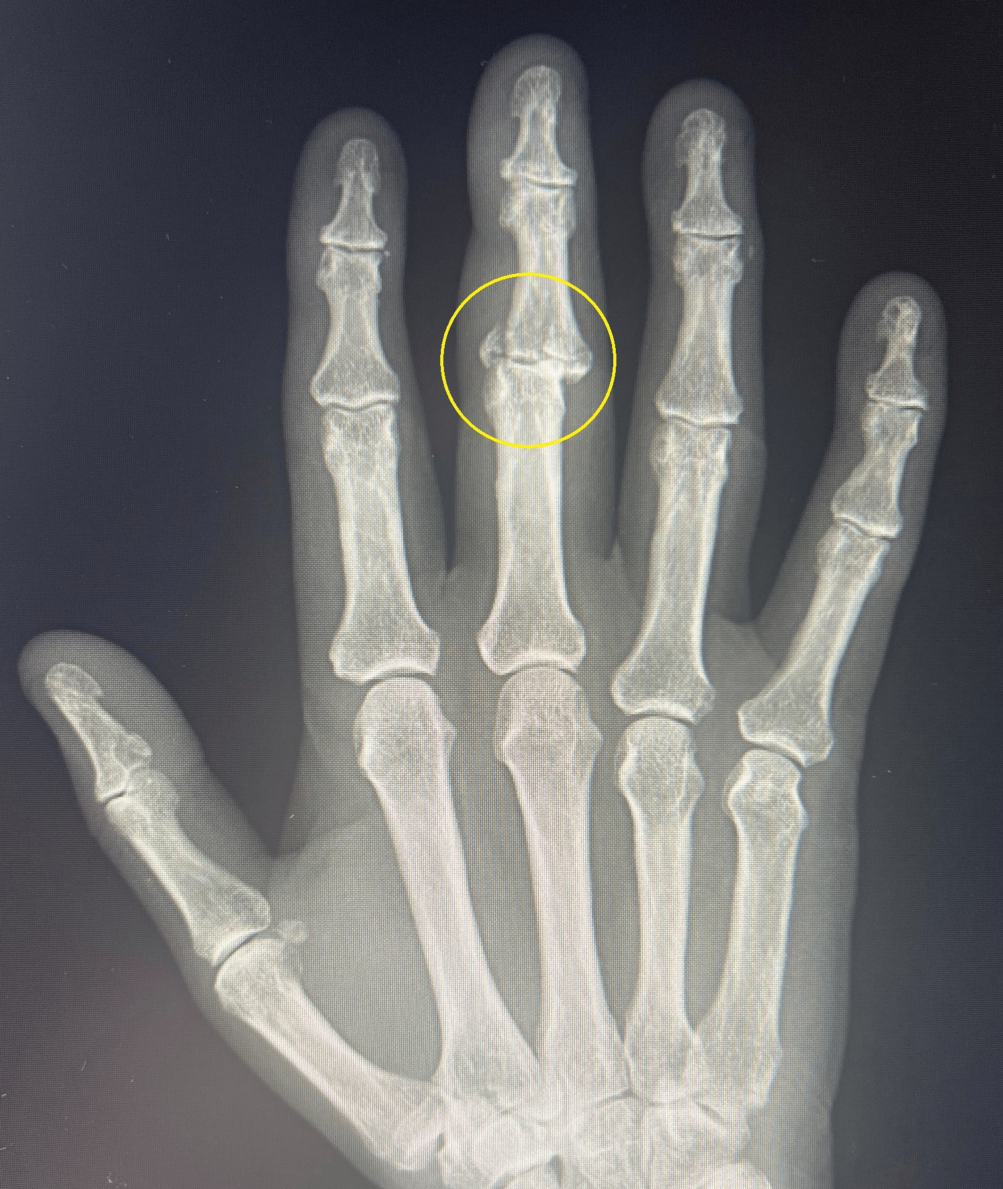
Comminuted pilon fracture of the PIP joint (X-ray in the AP projection) PIP: proximal interphalangeal
